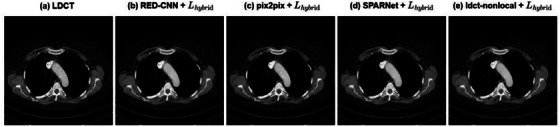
Denoising effect of each model using hybrid loss function on LDCT image of the external testing dataset. The images from (a) to (e) are LDCT image, and the denoising results of RED‐CNN, pix2pix, SPARNet, and ldct‐nonlocal using hybrid loss function, respectively. The CT display window is [−160 240] HU. It can be seen that each model shows a good denoising effect.
